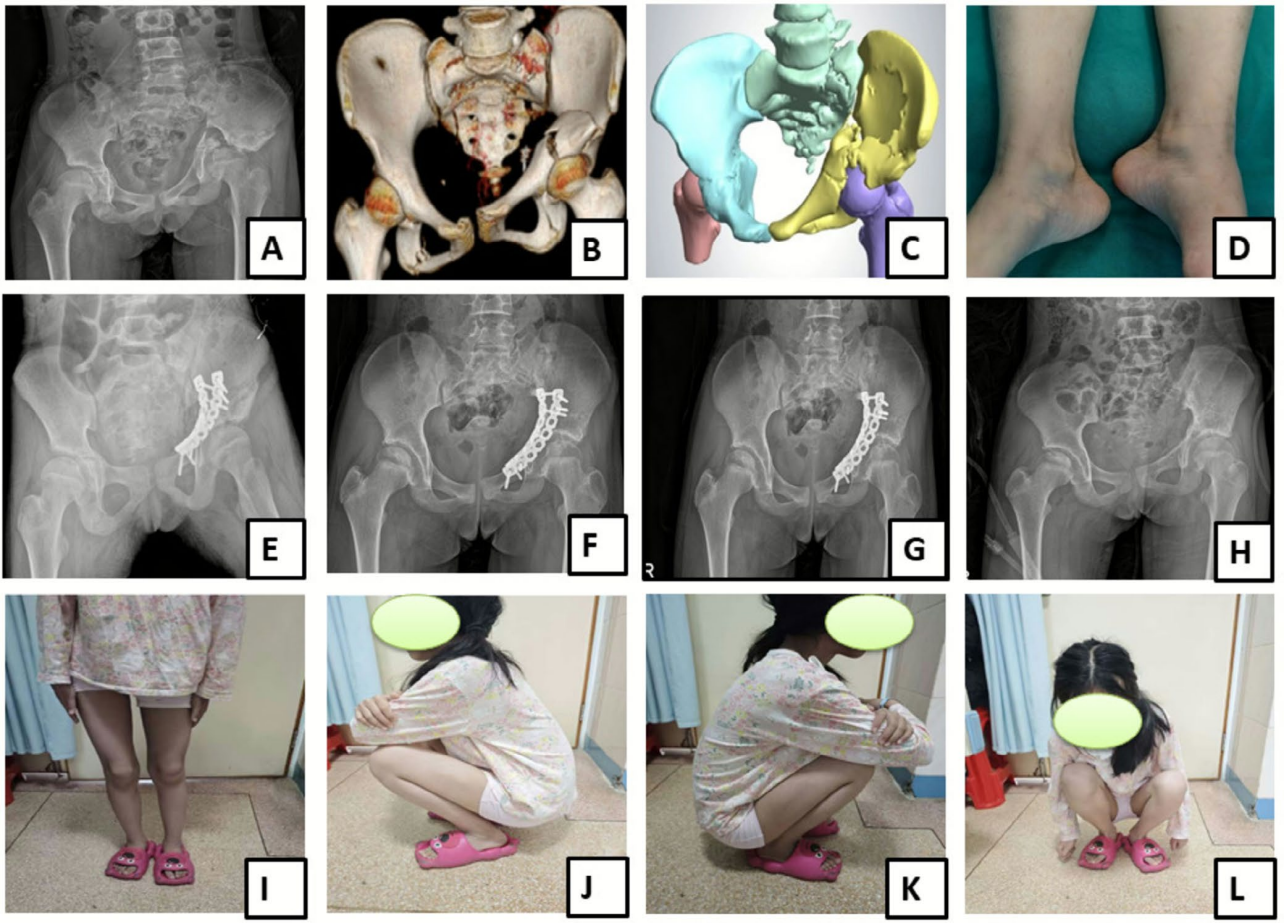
A 14‐year‐old female patient, admitted 6 weeks post‐injury of the right acetabulum following a fall from height. Post‐traumatic X‐Ray (A) and 3D CT scan (B) reconstruction image, describing the fracture lines on AP view; 3D reconstructed images (C) revealing a tilted left iliac bone with a fracture of both columns of the left acetabulum; Gross image (D) shows the discrepancy in limb length with the left leg shortened by about 3 cm; Postoperative radiographs at 1 month (E), 6 months (F), and 12 months (G) demonstrating normal fracture healing and restored joint congruence; Postoperative image (H) taken after internal fixation removal at 1 year, following complete healing; Postoperative functional outcome images (I, J, K, and L) after internal fixation removal.
